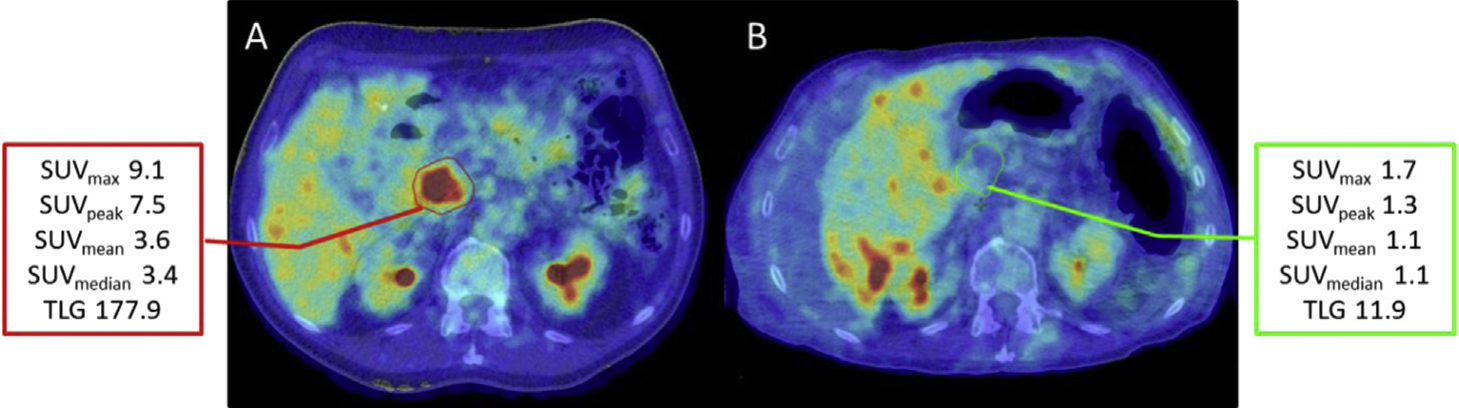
Transaxial images from pre- and post-chemoradiotherapy (CRT) ^18^F-fluorodeoxyglucose positron emission tomography/computed tomography (FDG-PET/CT) in a patient from the ARC2 study. Considerable changes can be seen from pre-CRT (A) and post-CRT (B). FDG avidity has decreased with a reduction in all of the PET parameters. The pre-CRT tumour region of interest (red) had to be modified on the post-CRT image (green). This is because the patient had developed ascites, which displaced the tumour and the liver. In addition, there was evidence of tumour shrinkage on the CT component of the PET/CT.
